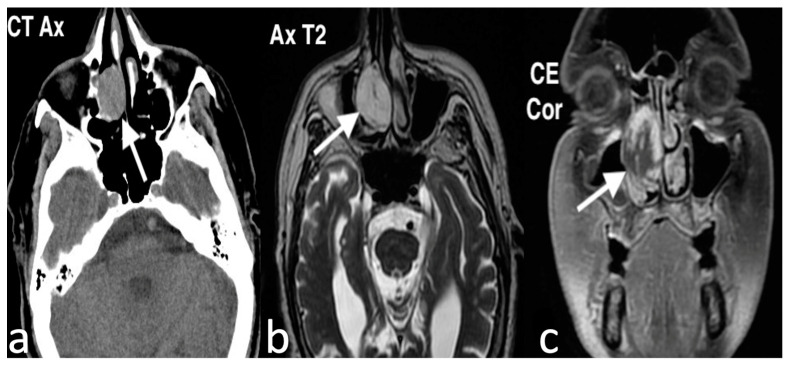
Hemangioma in a patient with right nasal obstruction and epistaxis. During rhinoscopy, a smooth dark-red mass was noted. The axial CT image (a) shows a well-defined, oval mass in the upper third of the right nasal cavity (arrow); on MR, the mass (arrow) occupies the middle–upper third of the right nasal cavity, showing high intensity on the axial T2 image (b); on coronal fat saturation T1 after intravenous Gd (c), the mass (arrow) shows intense heterogeneous enhancement.
